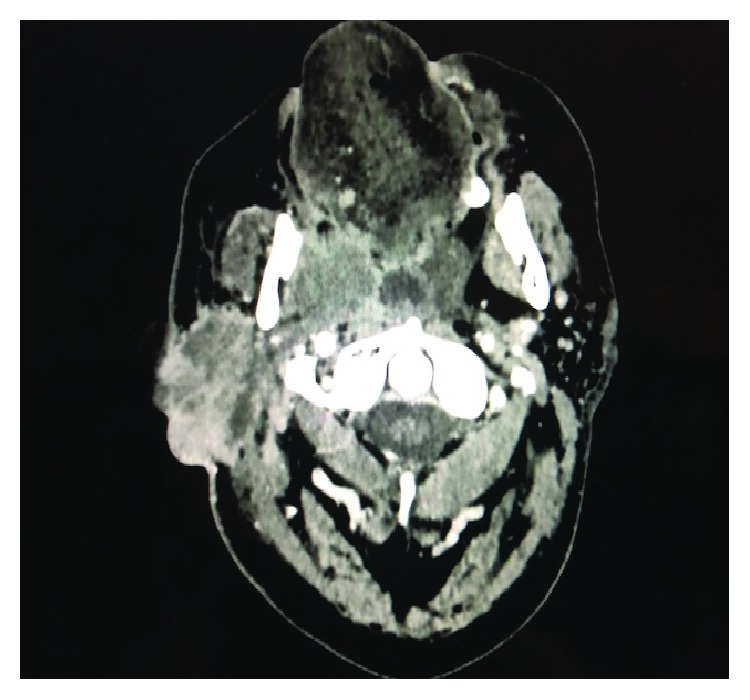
CT image of the rapidly growing tumor when the treatment was first interrupted.
